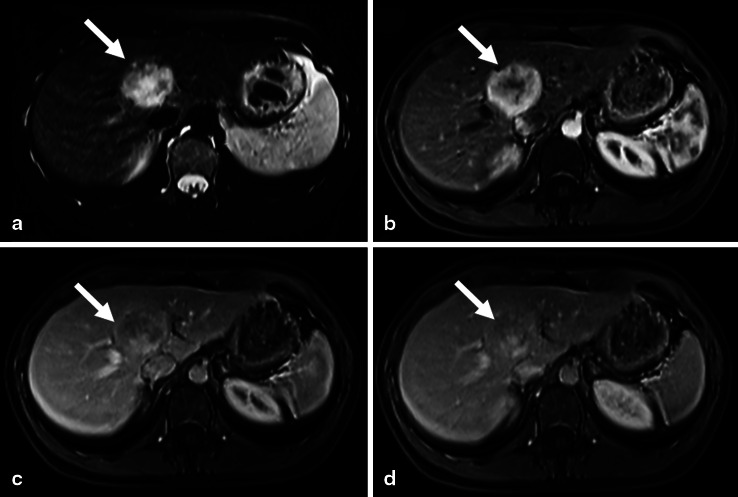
MRI: T2W image demonstrates a large, well-circumscribed hyperintense mass (a). Post-contrast T1W images showed highly heterogenous arterial enhancement, the central hyperintense area probably represents necrosis (b). In portal venous (c) and delayed phases (d), the lesion is iso- to hypointense to liver parenchyma with the central portion remaining almost nonenhanced.
